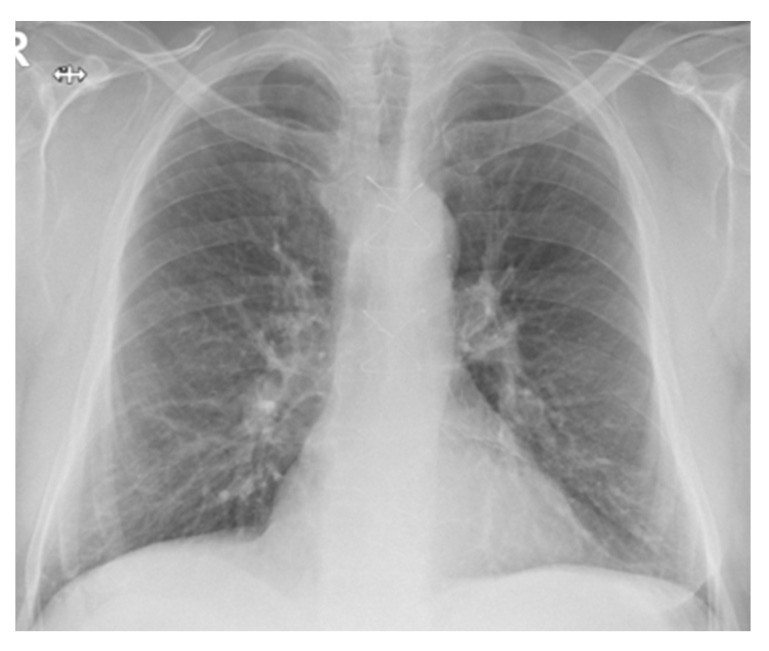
Chest X-ray—2022—Case 1. Diffuse reticular opacities, especially in the basal regions.
